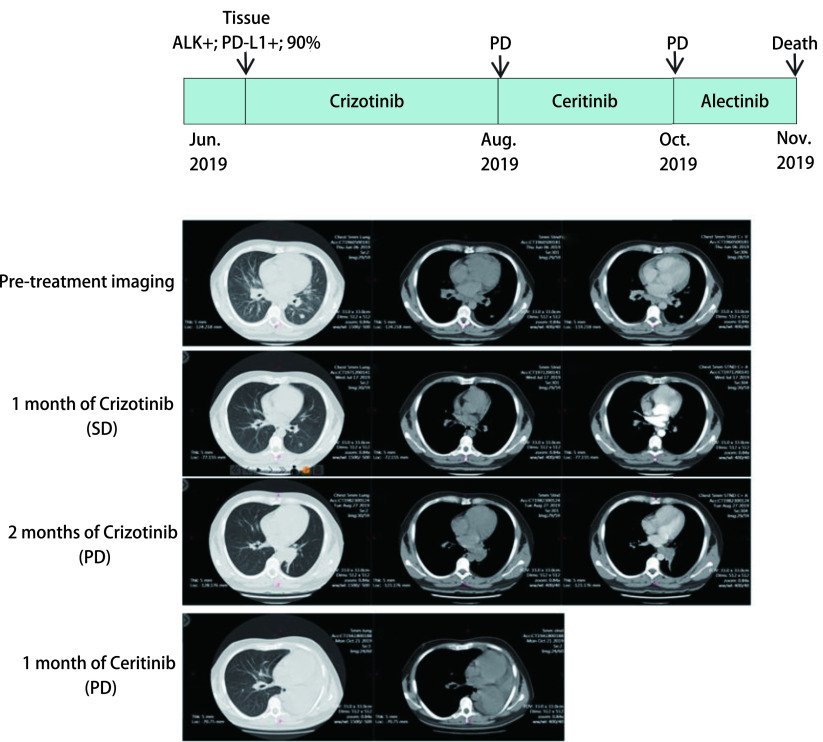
患者1治疗过程以及治疗前后CT诊断图 Timeline of patient evolution and chest computed tomography scans at diagnosis and after treatment in case 1. CT: computed tomography; PD: progressive disease; SD: stable disease.
